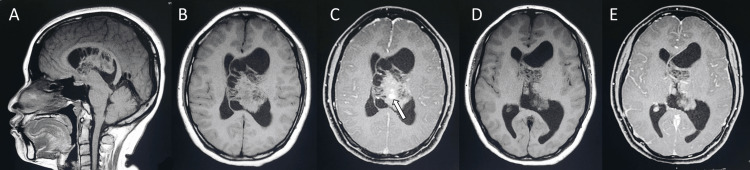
Magnetic resonance T1WI of the brain. (A) Midsagittal plane; (B) Axial plane at the lateral ventricle level; (C) Axial plane with gadolinium at the lateral ventricle level; (D) Axial plane at the third ventricle level; (E) Axial plane with gadolinium at the third ventricle level. The mass is isointense with mild to moderate heterogeneous enhancement (arrow). Obstructive hydrocephalus and pressure effects on the left thalamus are prominent. T1WI: T1-weighted image
